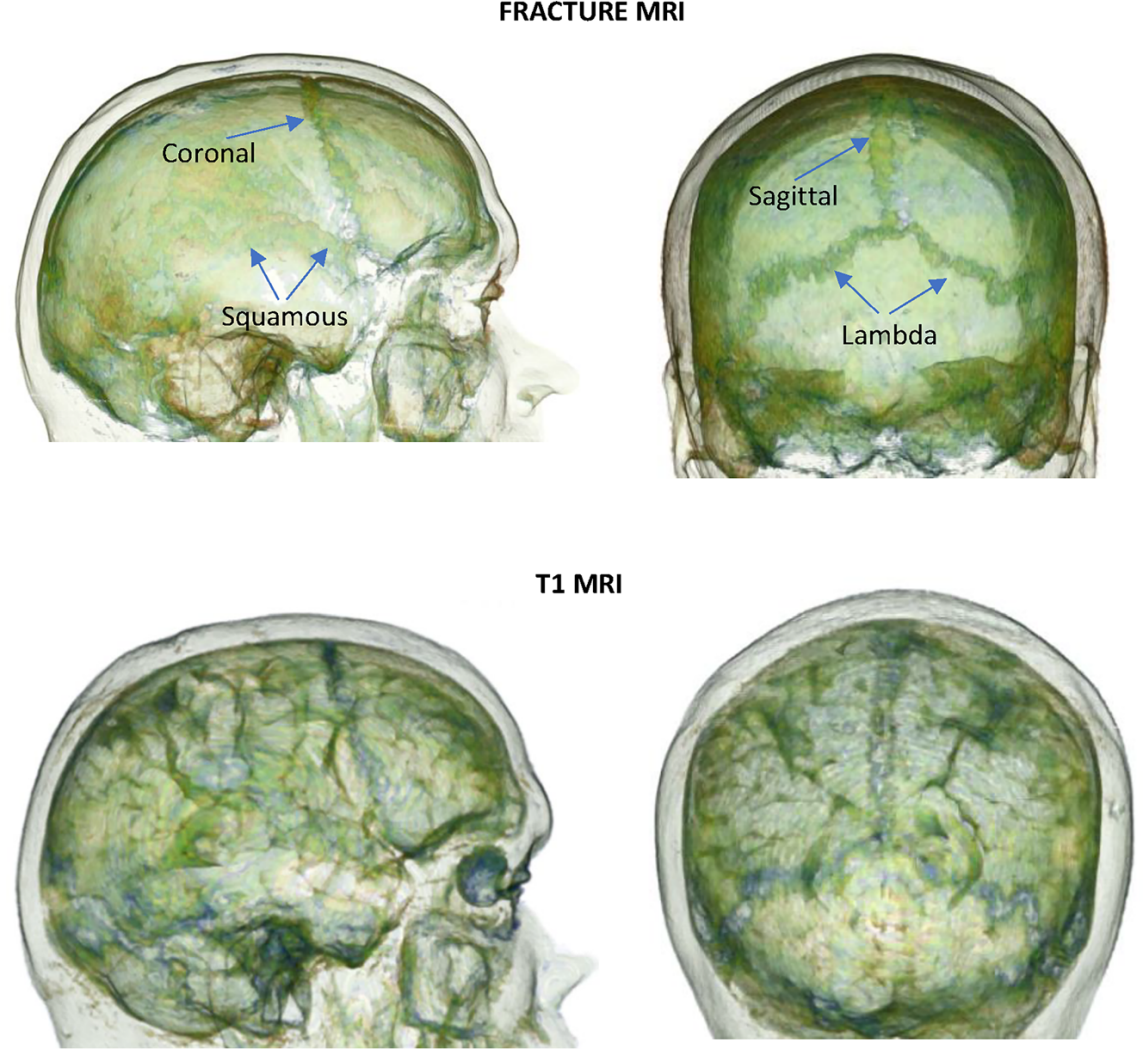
Volumetric rendering of T1 and FRACTURE MRI scan images—DICOM images of 45-year-old man (AC) uploaded to “3D Slicer v5.0.2” and volume rendering shift adjusted using the preset “CT-Lung” so the skull and sutures were visible. The sutures are clearly demonstrated in relation to soft tissue landmarks. HREC number HC190222 (research MRI scanning for protocol development).
